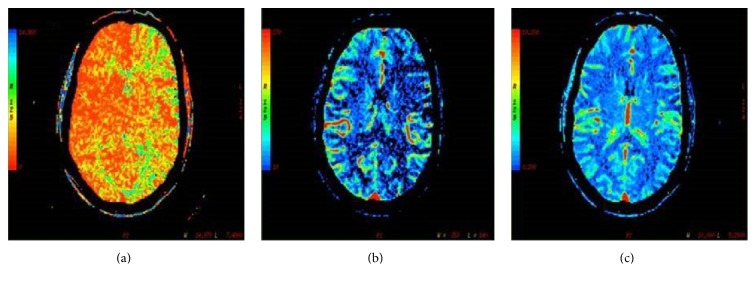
Perfusion computed tomography. TC revealed increased mean transit time (a) and diminished cerebral blood flow (b) with preserved cerebral volume (c). These changes were observed throughout the entire left cerebral hemisphere not confined to a particular vascular territory including the territory of anterior, middle, and posterior cerebral arteries (PCA).
